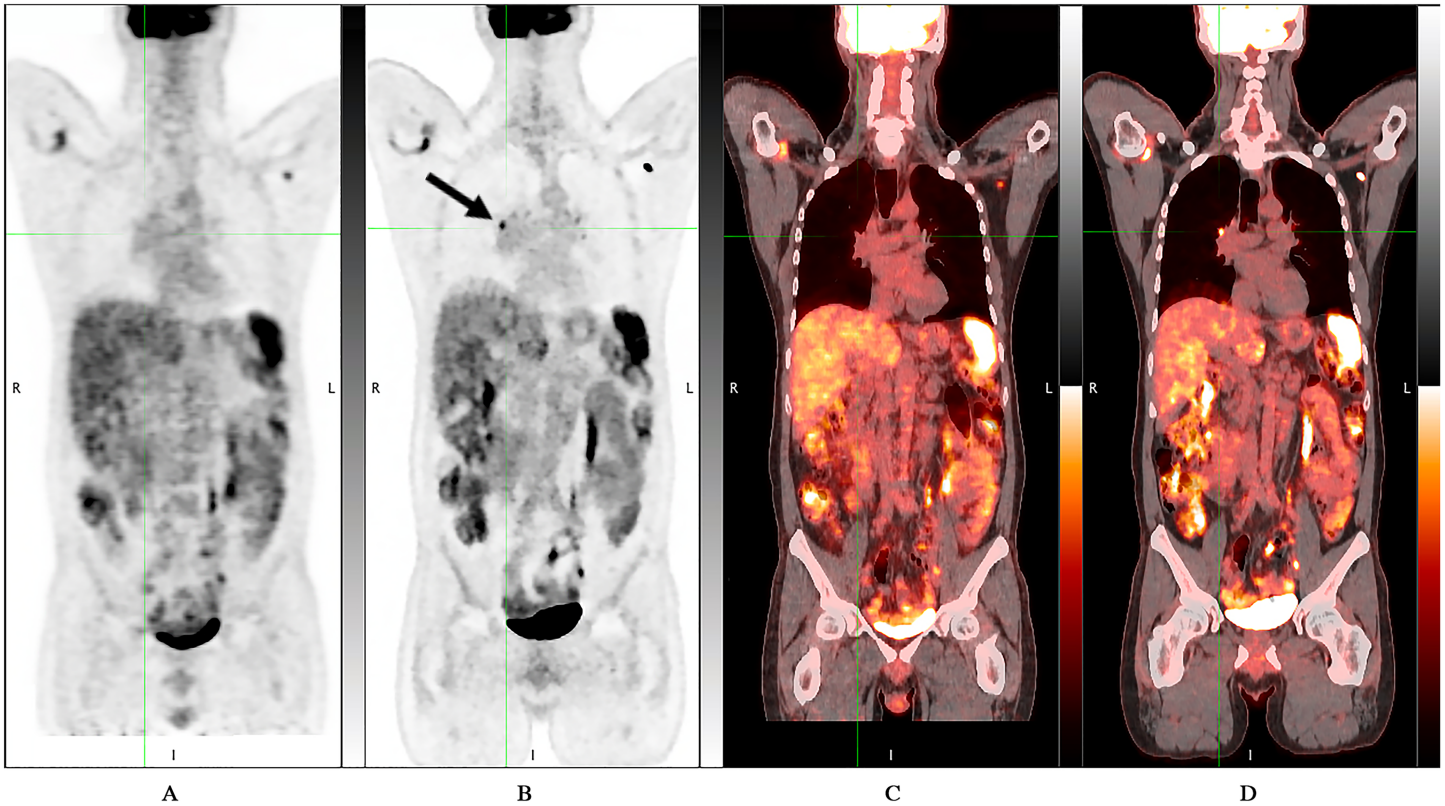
D690 PET/CT vs DMI PET/CT: DMI showed an additional focal avid FDG uptake area (black arrow). 46 years-old man with myocardial sarcoidosis who underwent PET/CT for initial staging. DMI images were acquired 39.1 minutes after standard acquisition and 109.2 minutes after ^18^F-FDG injection. A) D690 Maximum-intensity-projection (MIP); B) DMI MIP; C) D690 coronal fused image D) DMI coronal fused image.
